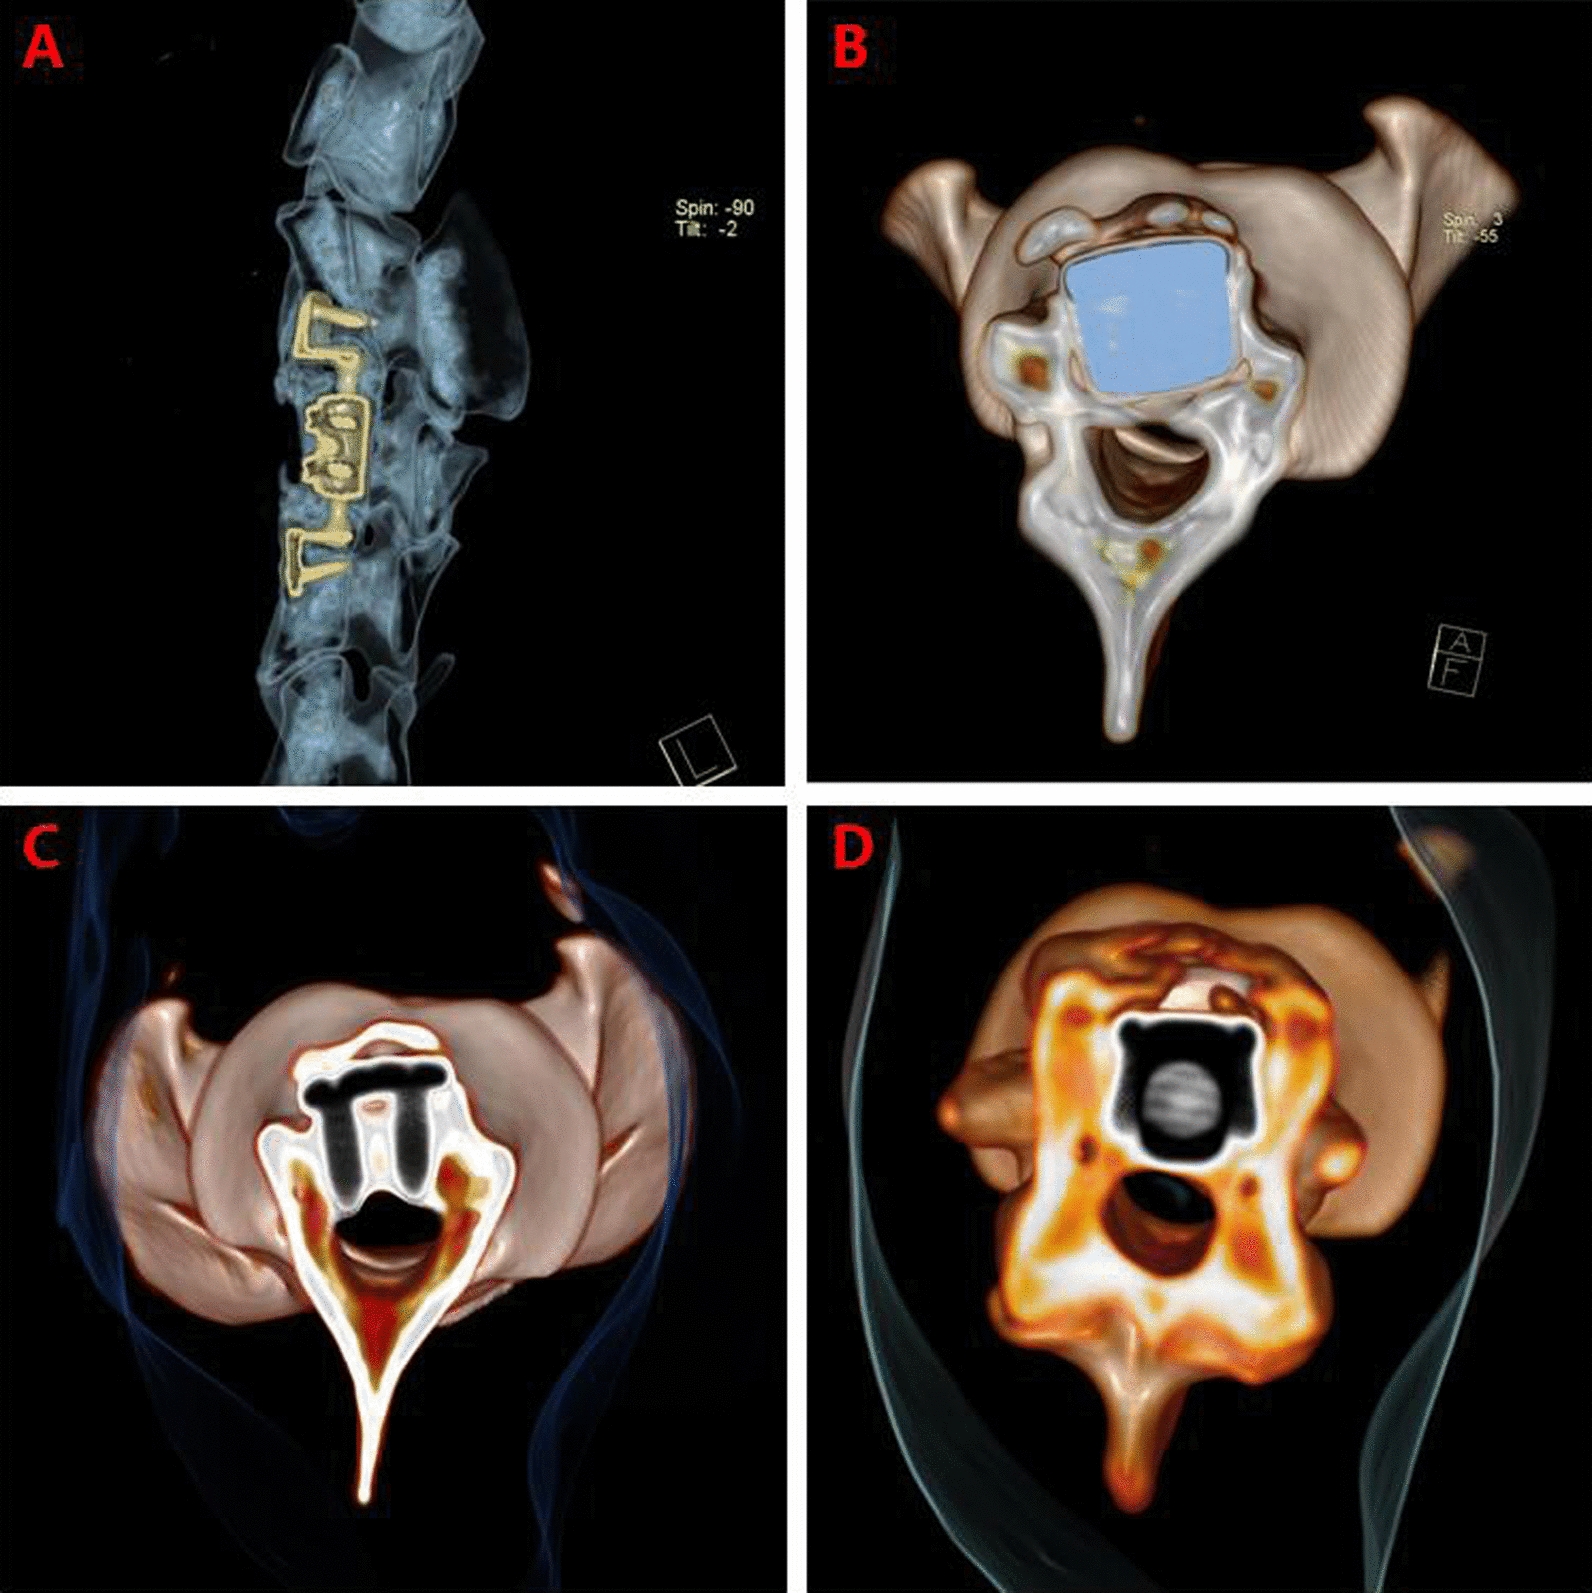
3D CT reconstruction6 months after surgery. A, Sagittal view. B, C, D, Axial view
